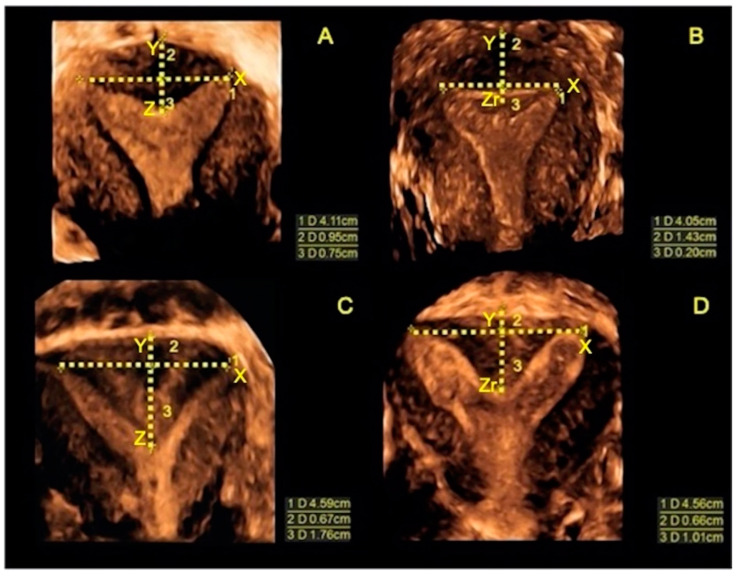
Ultrasound comparison between external and inner uterine profiles of septate uterus before surgery and three months after surgery. In each image, all the measurements of the septum are shown (the uterine wall thickness (Y), the base of the septum (X), the length of the septum before surgery (Z) and the length of the new inner uterine edge after surgery (Zr)). (A) Ultrasound midcoronal view of a partial septate uterus (U2a according to ESHRE/ ESGE) before surgery; (B) Ultrasound midocoronal image of the same uterus three months after surgery, characterized by the remodeling of the fundus; (C) Ultrasound midcoronal view of another partial septate uterus (U2a according to ESHRE/ ESGE) before surgery; (D) Ultrasound midcoronal image of the previous uterus three months after surgery, characterized by a residual partial septum. This patient was scheduled for a second- step metroplasty.
